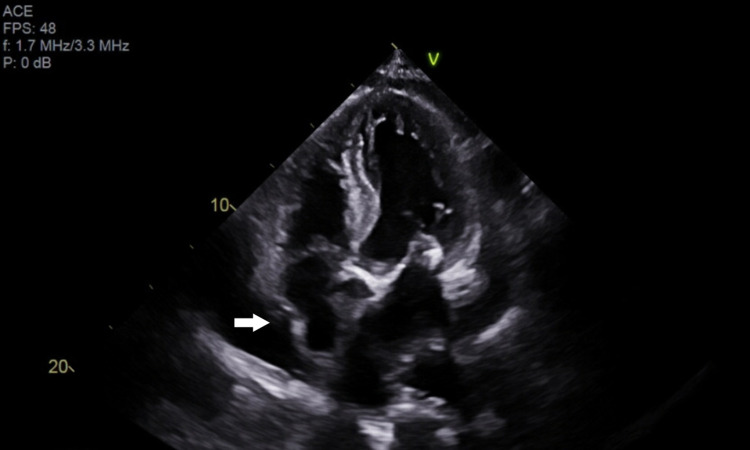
Apical four-chamber view of the heart on TTE showing inversion of the right atrium (a sign of tamponade). TTE: transthoracic echocardiography
